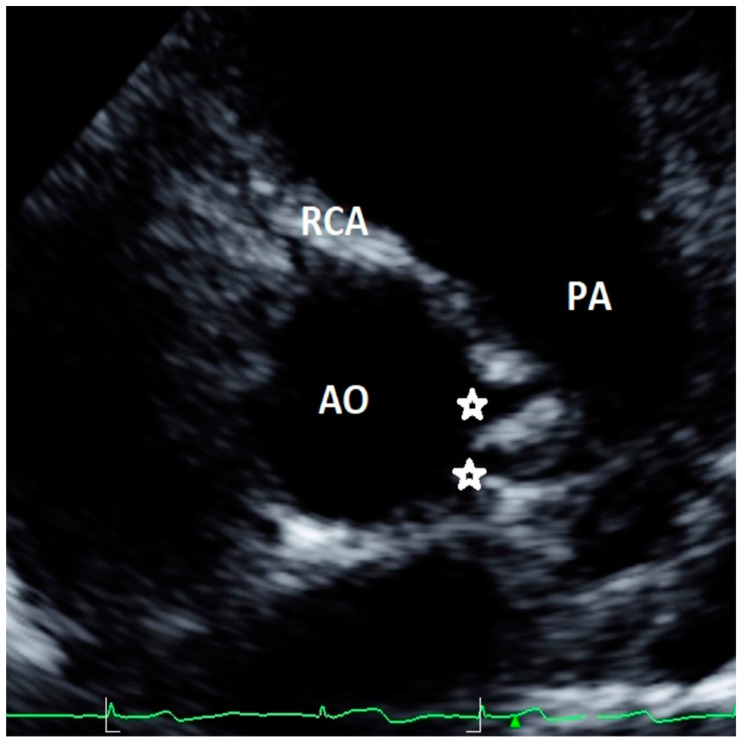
Asymptomatic 13-year-old boy evaluated with negative stress testing for murmur at preparticipation evaluation for sports competition. Parasternal short-axis view at the level of aortic root showing separate origins of circumflex (CFx) and left descending artery (LAD).
